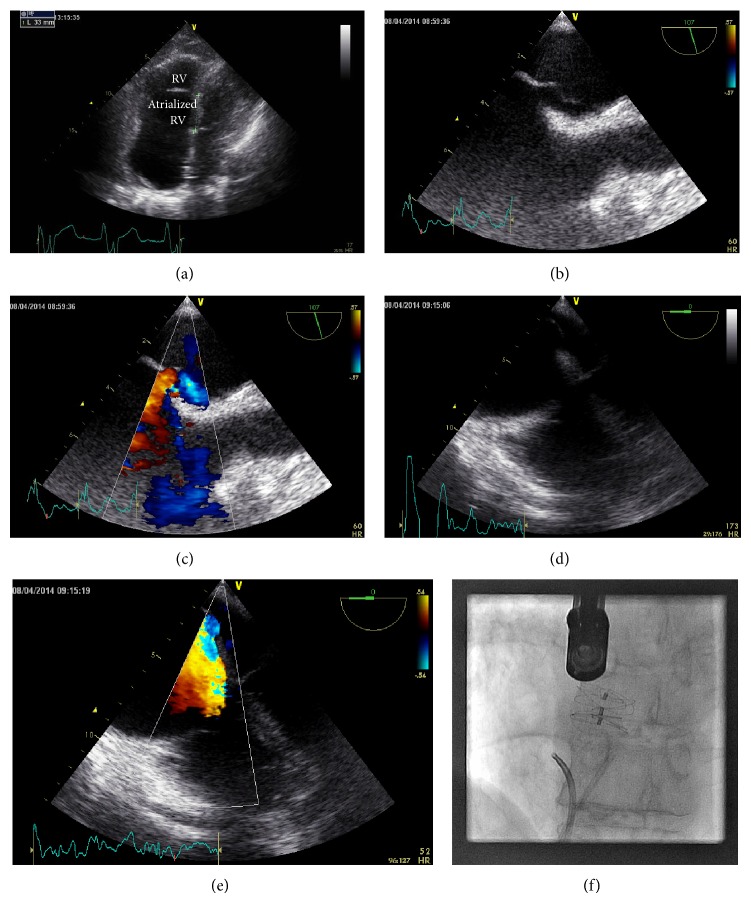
(a) TTE apical four-chamber view, focused on the right heart, showing Ebstein anomaly with atrialization of the right ventricle. (b) TEE image (107 degrees) showing the patent foramen ovale (arrow). (c) TEE image (107 degrees) with color Doppler, showing the patent foramen ovale. (d) TEE four-chamber view (0 degrees) showing the tricuspid valve and the patent foramen ovale. (e) TEE four-chamber view (0 degrees) with color Doppler, showing the eccentric tricuspid regurgitation jet flowing through the patent foramen ovale. (f) Coronary angiogram showing a 20 mm Gore Septal Occluder device used for partial percutaneous patent foramen ovate closure.
